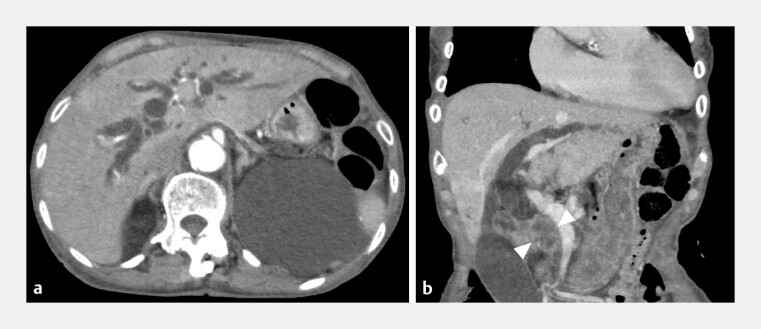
Computed tomography images show pancreatic head cancer (arrowheads) and dilated intrahepatic bile duct.
